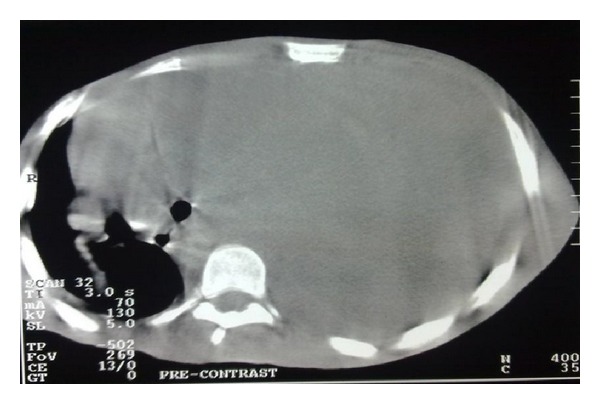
Chest CT scan showing non-enhancing mass, isodense to surrounding muscle completely occupying the entire left hemithorax.
